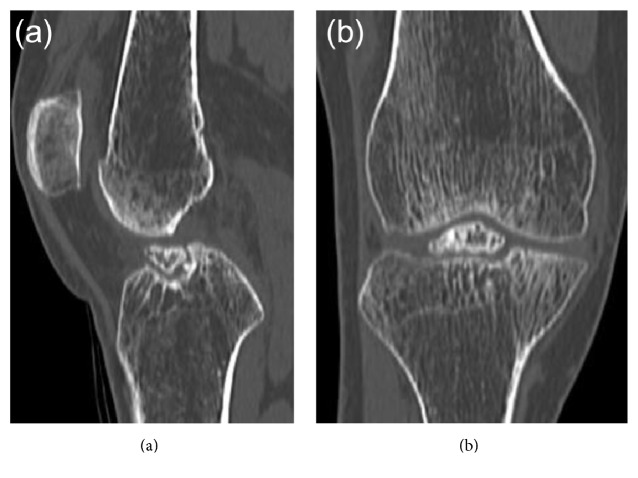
Preoperative computed tomography of our patient. (a) Sagittal and (b) coronal views, showing the avulsed fragment at the anterior tibial eminence of the right knee impinging on the femoral intercondylar notch.
